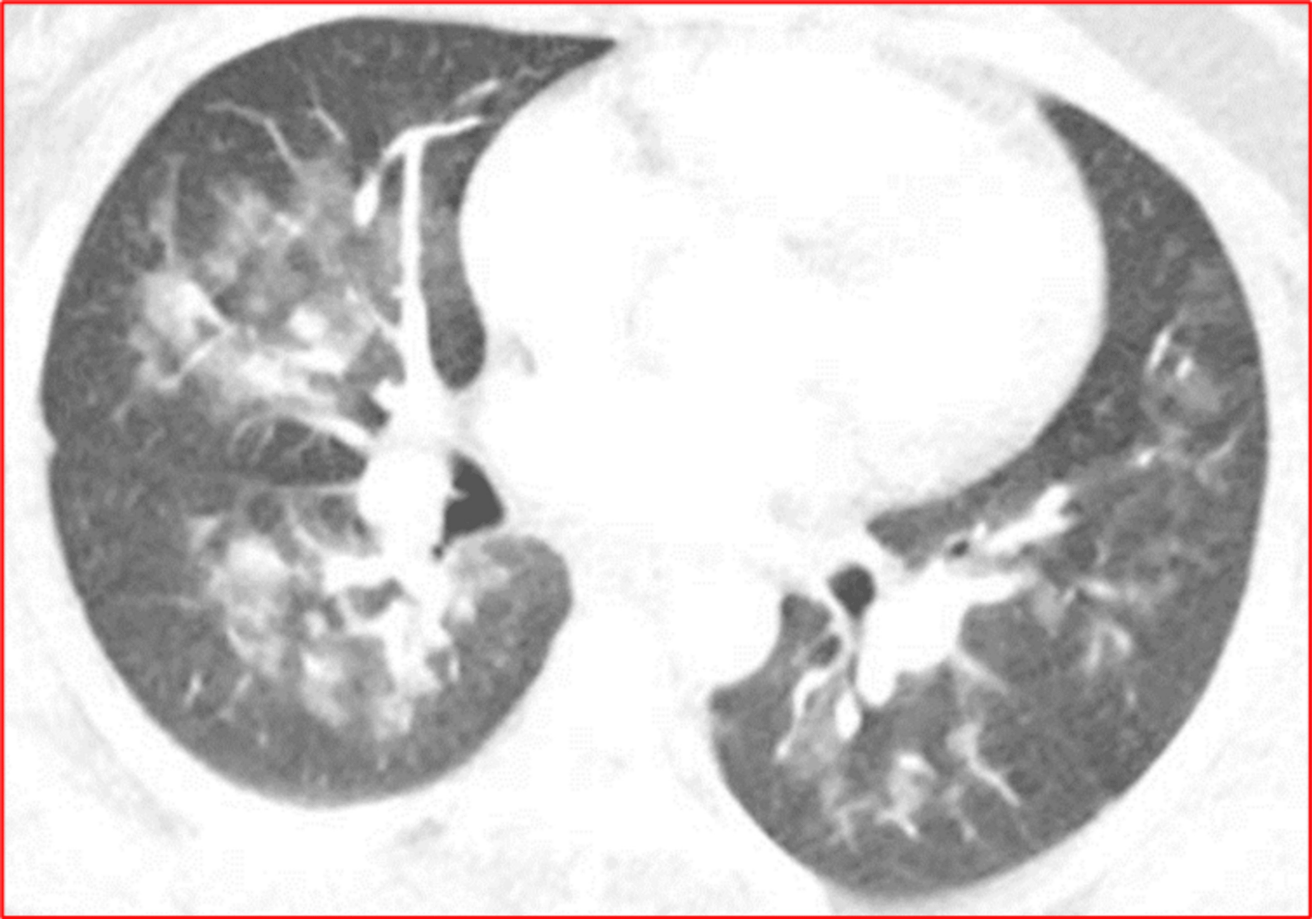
Typical CT chest findings in FES showing bilateral alveolar opacities. This figure was obtained from Dr. Nissar Shaikh’s image collection; the patient's informed consent was obtained. FES: fat embolism syndrome
